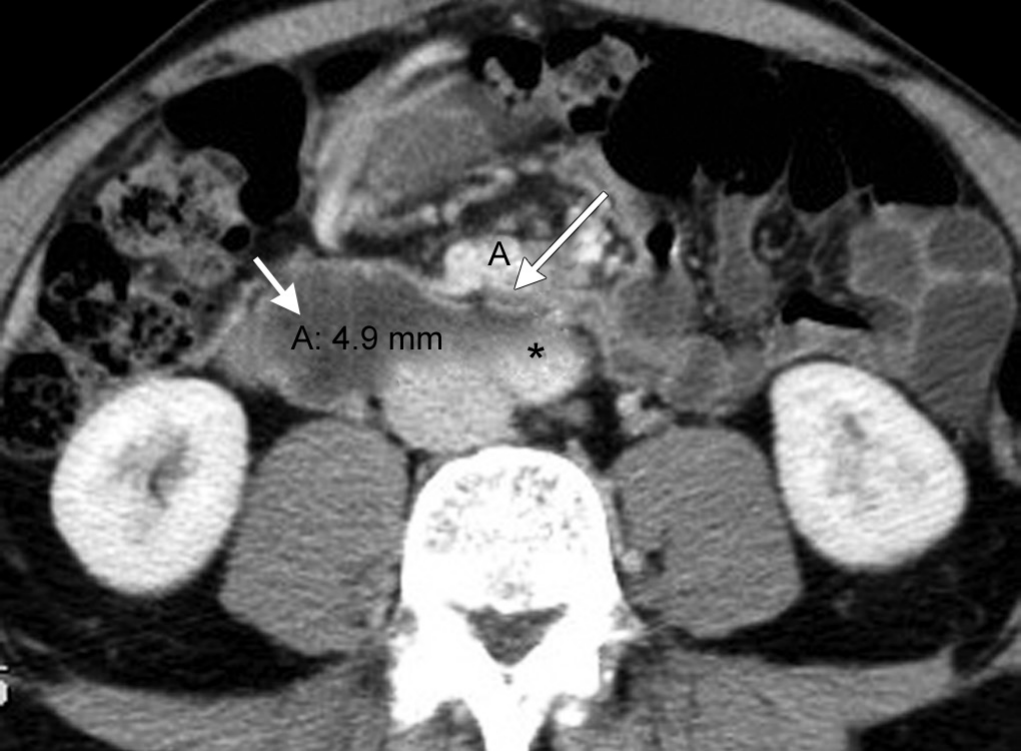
Axial CT scanning of the same patient shows markedly reduced AMD of about 5 mm between SMA (long arrow) and aorta (*) with distension of proximal duodenum (short arrow)
